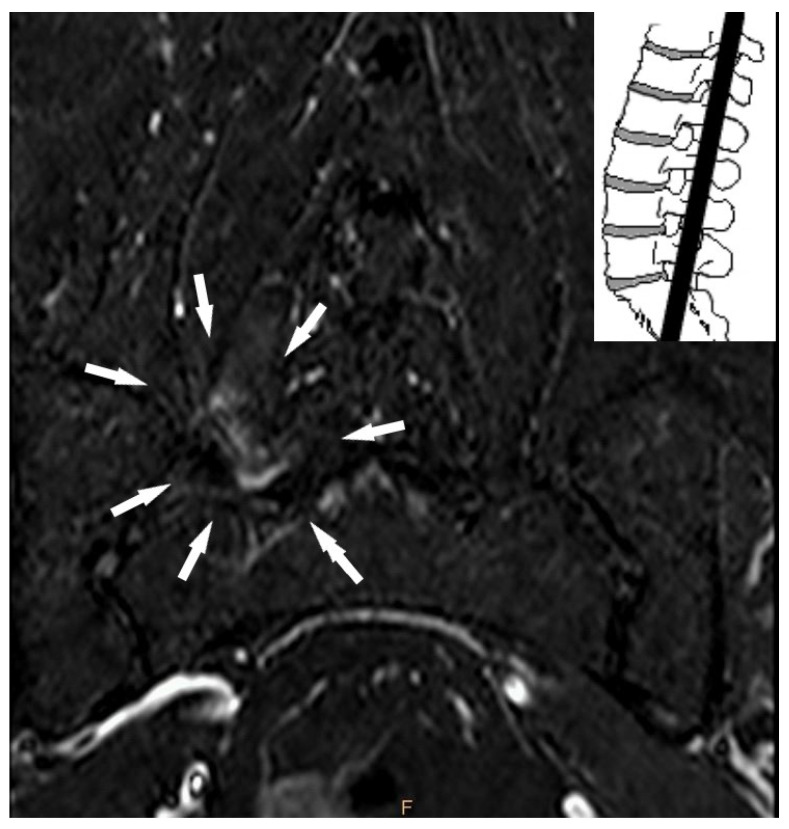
Axial short tau inversion recovery (STIR) T2-weighted sequence showing right L5-S1 facet joint bone marrow edema signal (white arrows).
